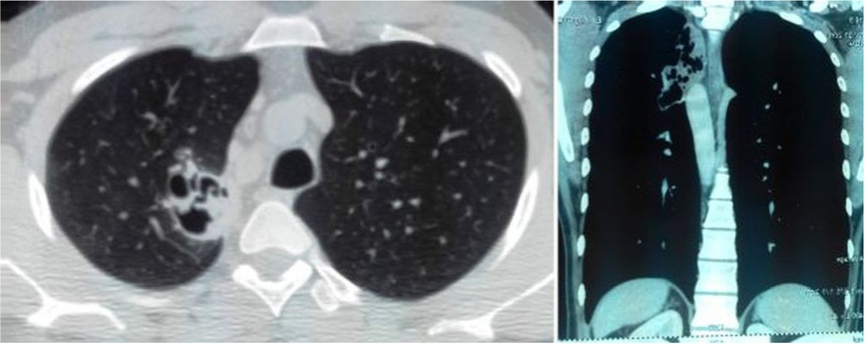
Contrast enhanced computed tomograph of chest. Contrast Enhanced Computed Tomograph of chest showing an irregular moderately thick walled cavitatory lesion in the left upper lobe postero-medially with nodularity along the inner wall of the cavity.
